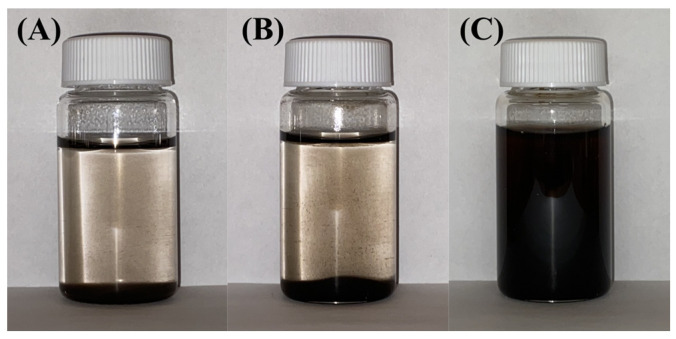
Fe_3_O_4_ suspension in water with (A) no dispersing agent, (B) BA as a dispersing agent, and (C) DHBA as a dispersing agent 1 h after ultrasonication.
